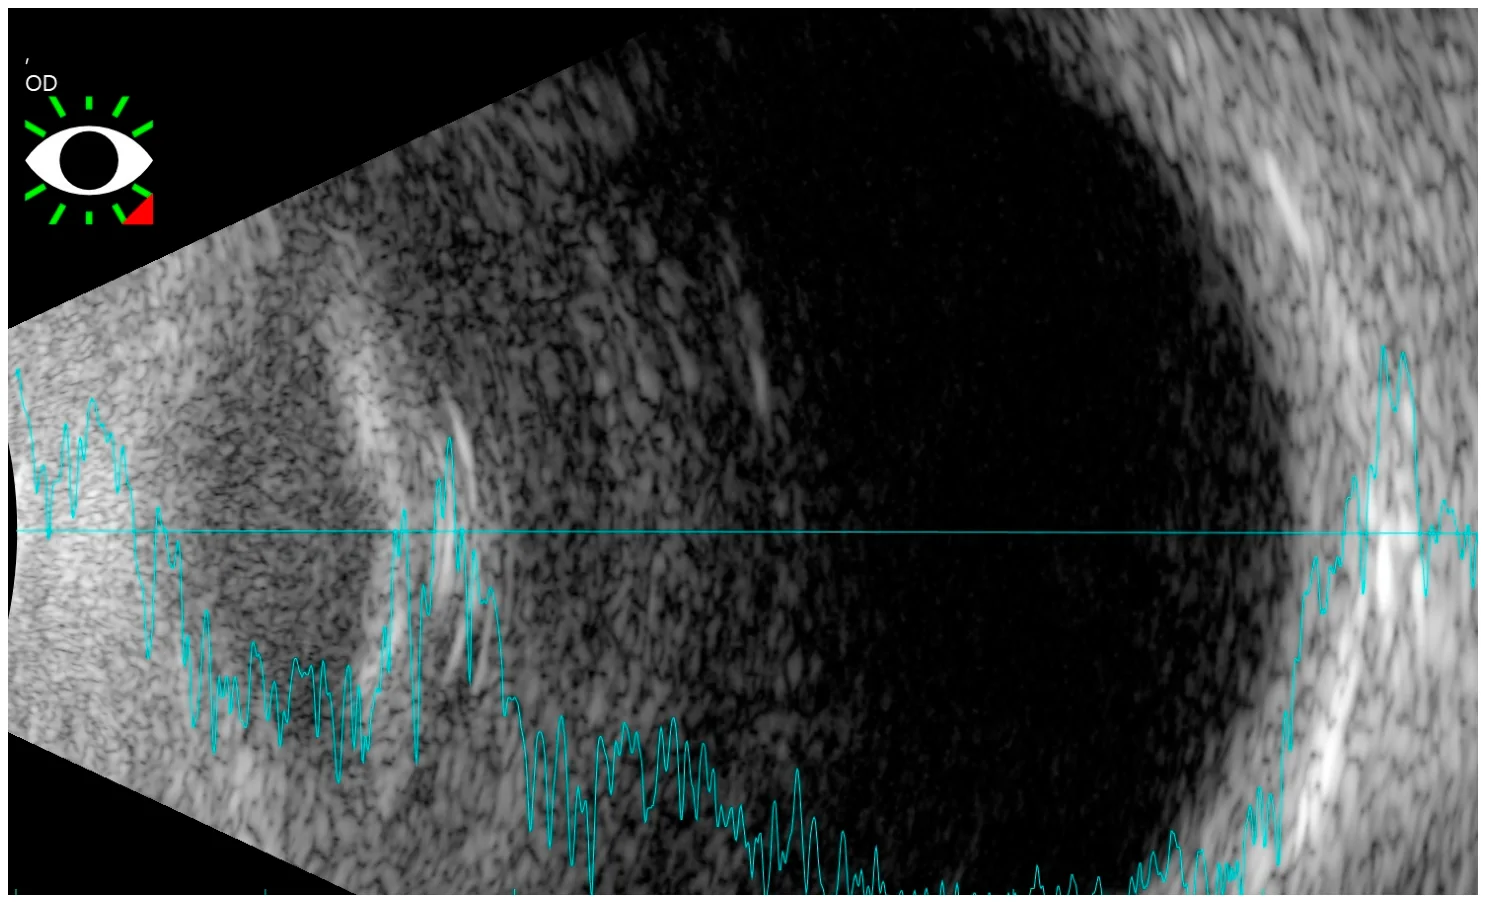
Conventional A- and B-scan ultrasonography of the right eye. The examination localized the posteriorly dislocated lens and showed no retinal detachment or intraocular mass. It was not used to assess capsular integrity or anterior-chamber angle anatomy.
